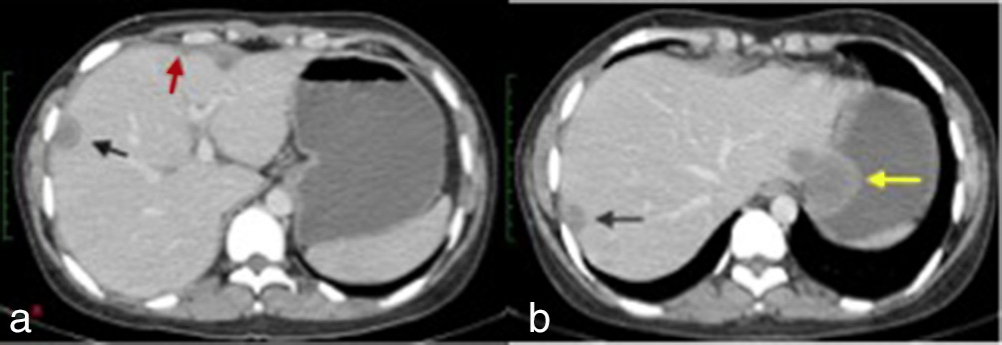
(a, b) Axial contrast-enhanced CT images of the abdomen showing multiple well-circumscribed, hypodense subcapsular lesions (black arrows) in the periphery of the liver with adjacent capsular thickening (red arrow), leading to the “frosted” liver appearance. The largest subcapsular lesion indents are on the gastric fundus (yellow arrow).
